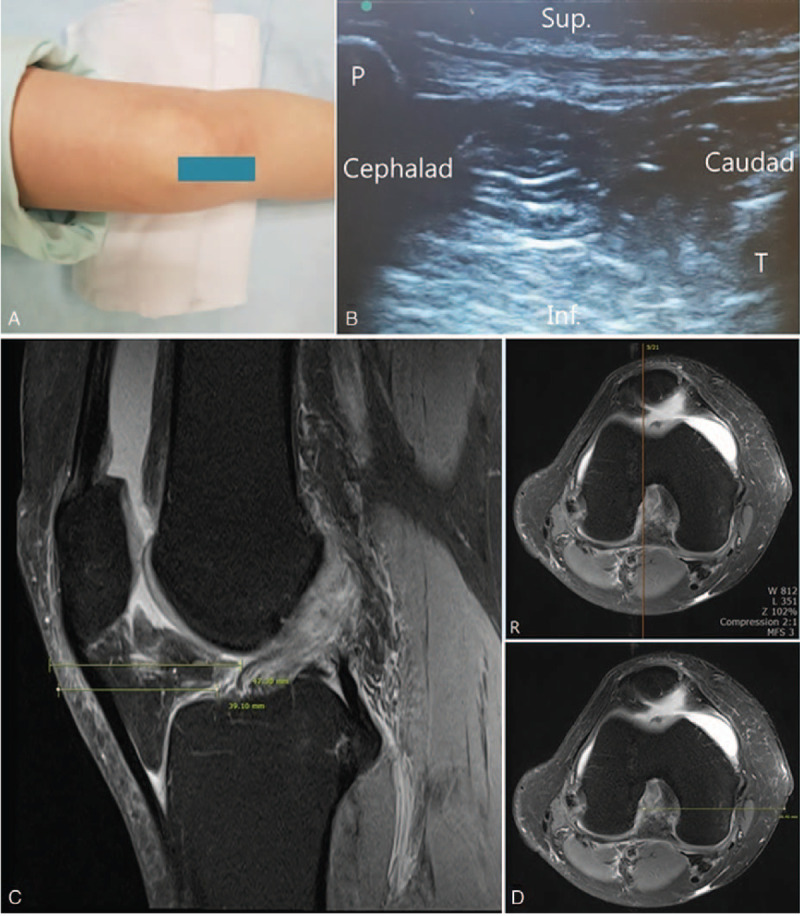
A) Ultrasound guided procedure of the knee: intraarticular injection. Longitudinal imaging over anterior knee.(square), B) Haziness of joint cavity, tibia (T), patella (P)., C) MRI knee Rt. (non-enhance axial view): Same level of the knee for measured a distance skin to target point., D) MRI knee Rt. (non-enhance sagittal view): Same level of the knee for measured a depth skin to target point. MRI = magnetic resonance imaging.
